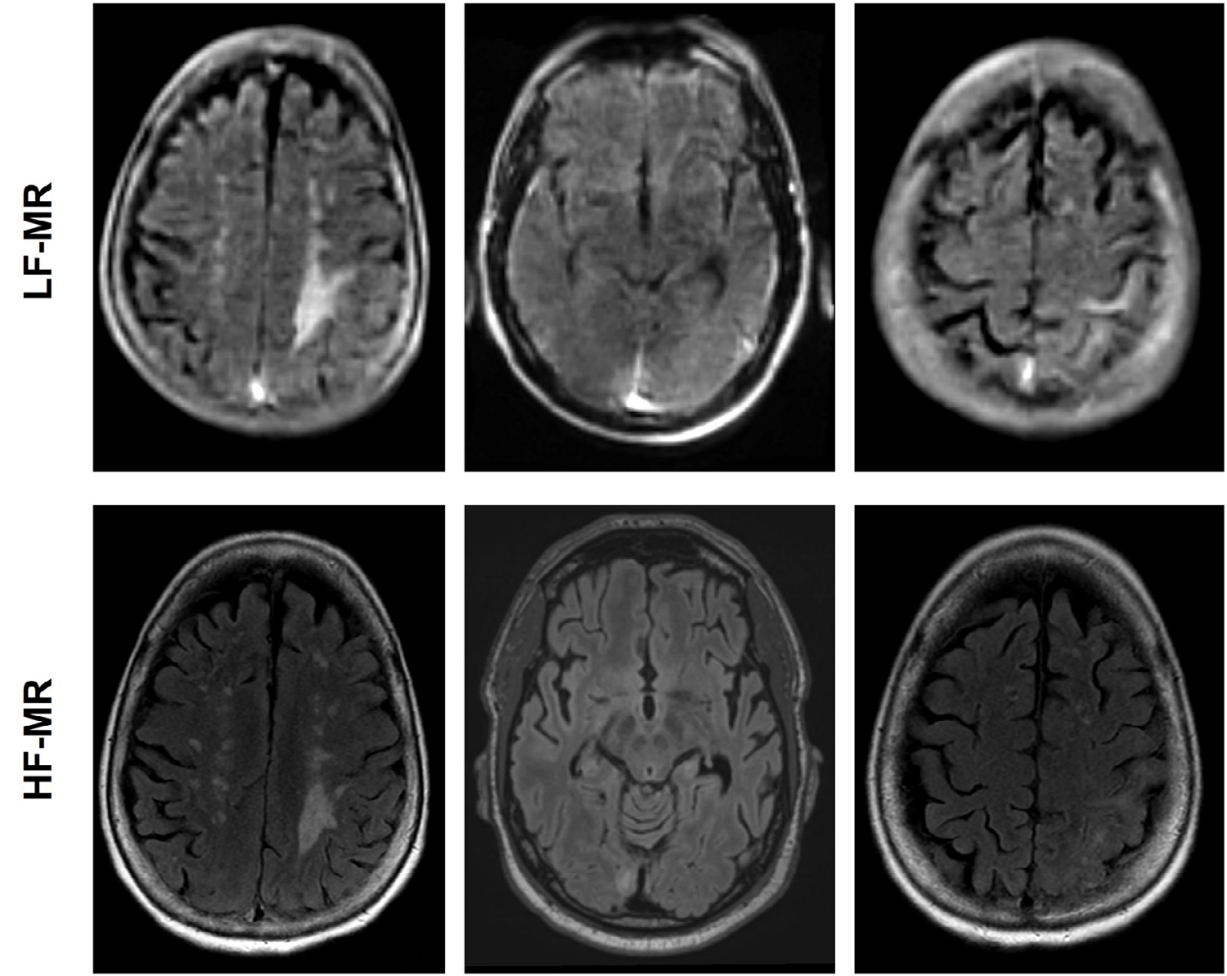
# Portable Low‐Field MRI for Rapid Detection and Monitoring of ARIA in Lecanemab‐Treated Alzheimer's Disease Patients: A Pilot Study
